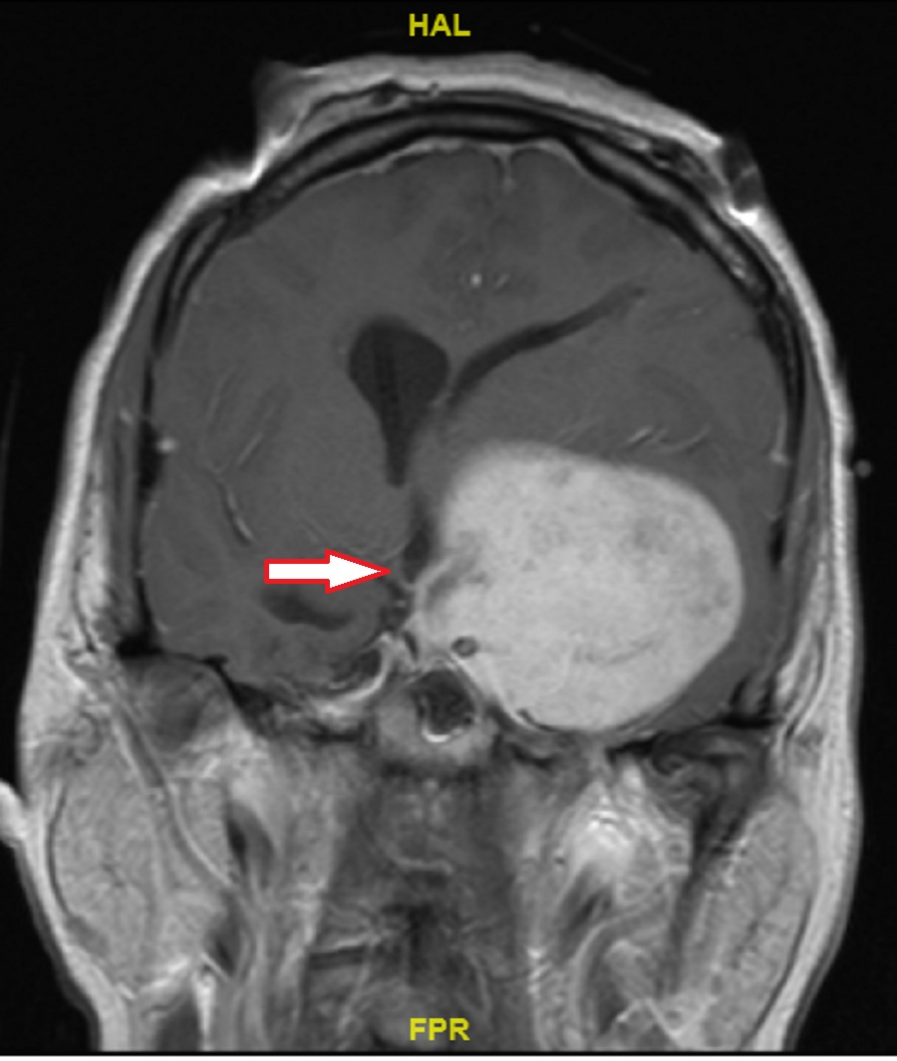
Initial T1 Contrast-enhanced Magnetic Resonance Imaging Coronal view of tumour (arrow)
